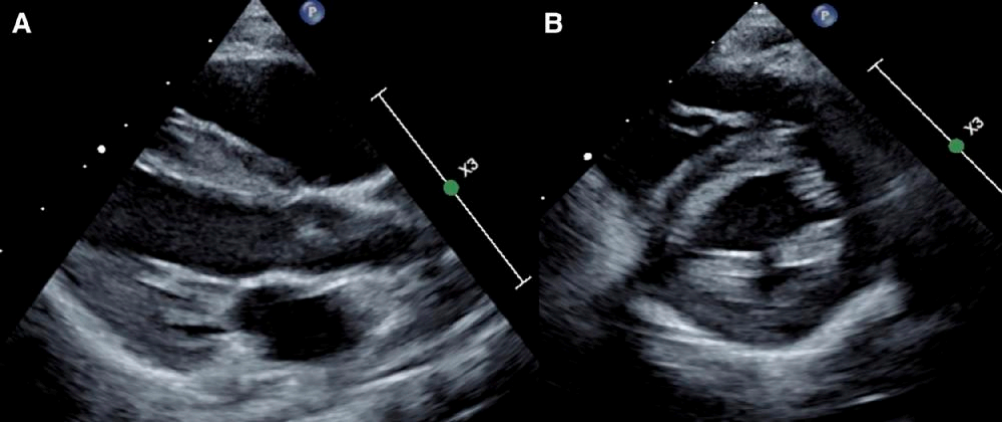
Two-dimensional parasternal long-axis (*A*) and short-axis (*B*) images at end-diastole demonstrating reduced left ventricular size after antegrade conduction via the accessory pathway was abolished with flecainide.
